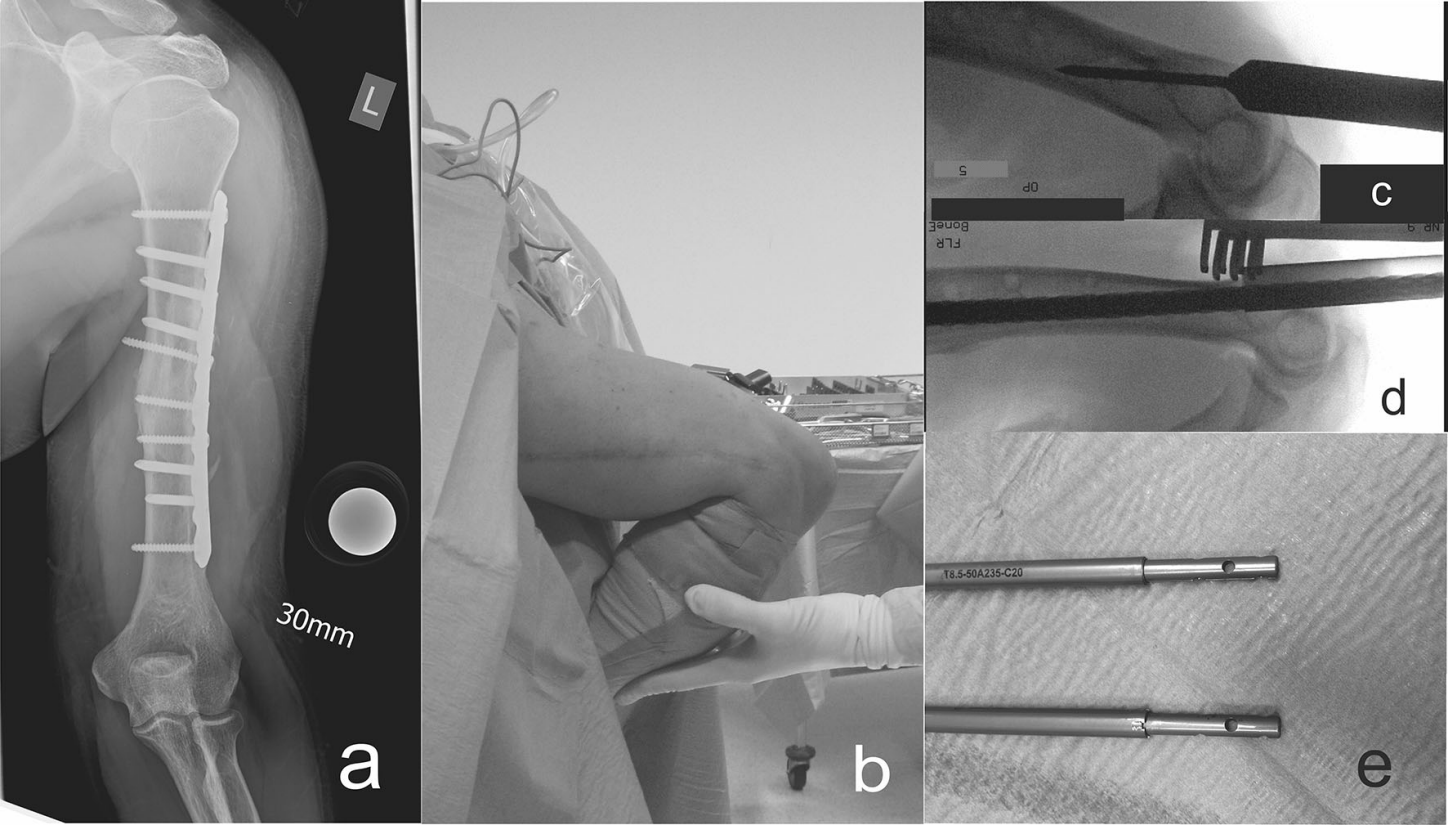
a Preoperative radiograph of patient G.K. with 40 mm shortening of the left humerus after a successfully treated nonunion; b intraoperative supine positioning of the patient for retrograde approach; c and d steel sleeves and rigid reamers for preparing the intramedullary canal; e crown breakage of the first PRECICE (P 2) below, predistracted nail above (P 2.1)
